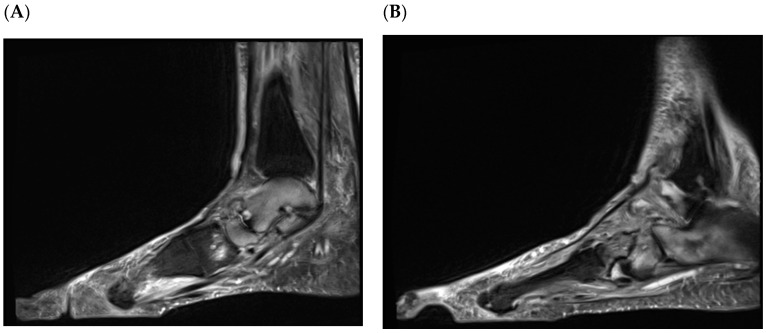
MRI scan of Subject 1 shows, in addition to talo-navicular bone and joint disruption (A), extensive bone marrow oedema in the calcaneocuboid region (B), which appears non-affected on X-ray (Figure 3D).
